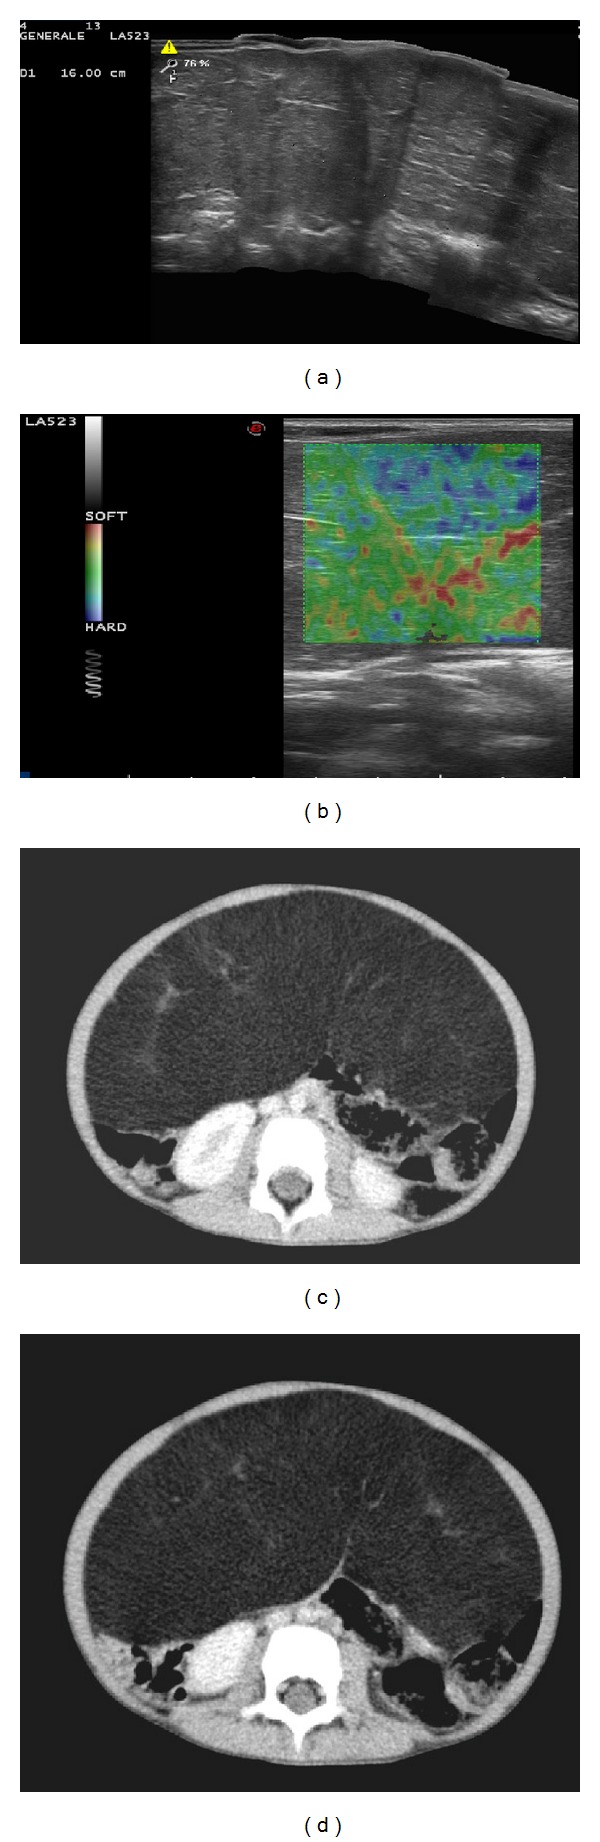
(a) US examination revealed mass had mixed echogenicity with some hyperechoic thin septations. (b) The mass appeared mainly green—soft—on elastosonography. ((c), (d)) CT showed the mass had fatty density with a few septations and no contrast enhancement.
